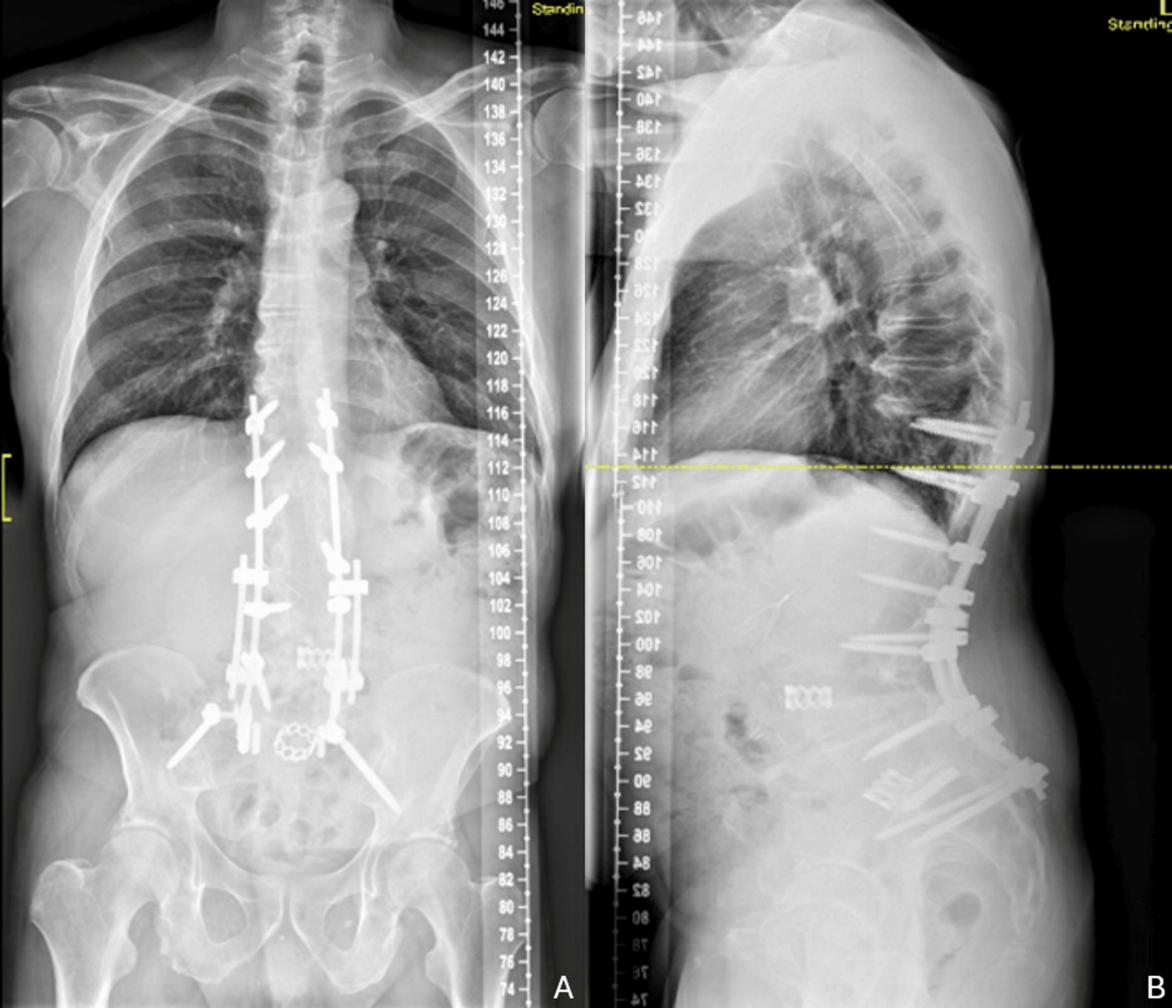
Postoperative lumbar images showing new construct after L4 PSO and revision posterior spinal fusion A: postoperative AP radiograph, B: postoperative lateral radiograph AP: anteroposterior, PSO: pedicle subtraction osteotomy
